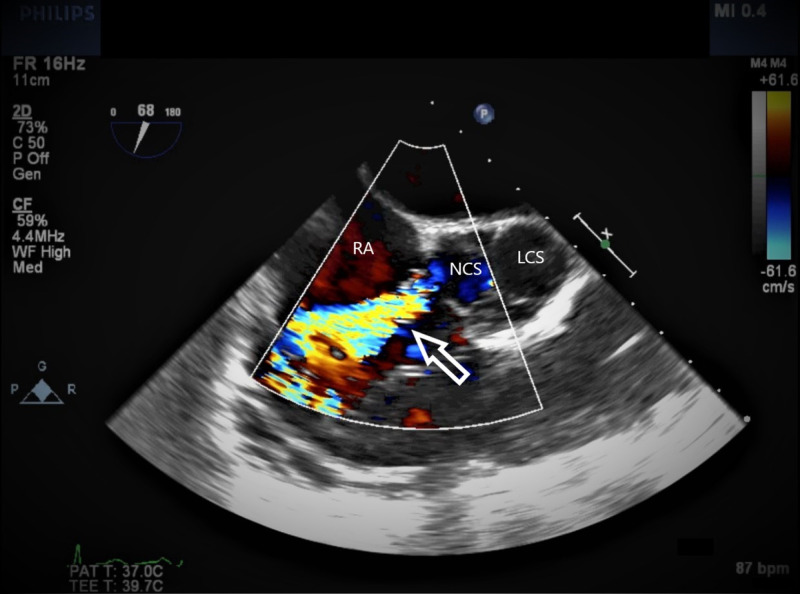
TEE with color Doppler The arrow indicating the high-velocity jet from the NCS into the RA through the ruptured SVA. TEE, transesophageal echocardiogram; SVA, sinus of Valsalva aneurysm; RA, right atrium; NCS, noncoronary sinus; LCS, left coronary sinus.
